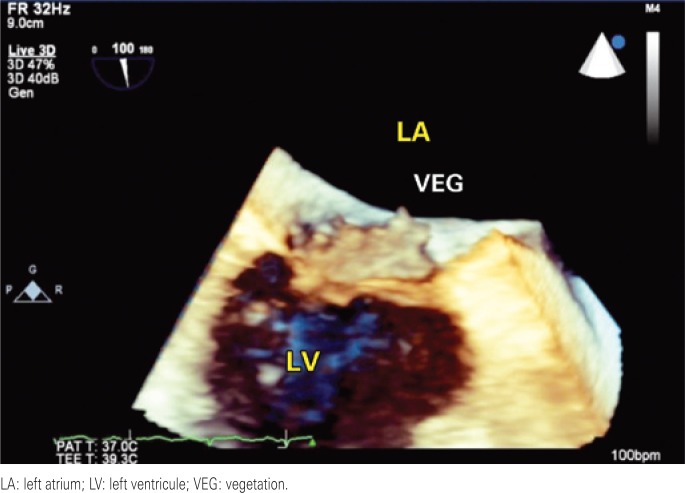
Three-dimensional transthoracic echocardiogram demonstrating mitral valve vegetation
